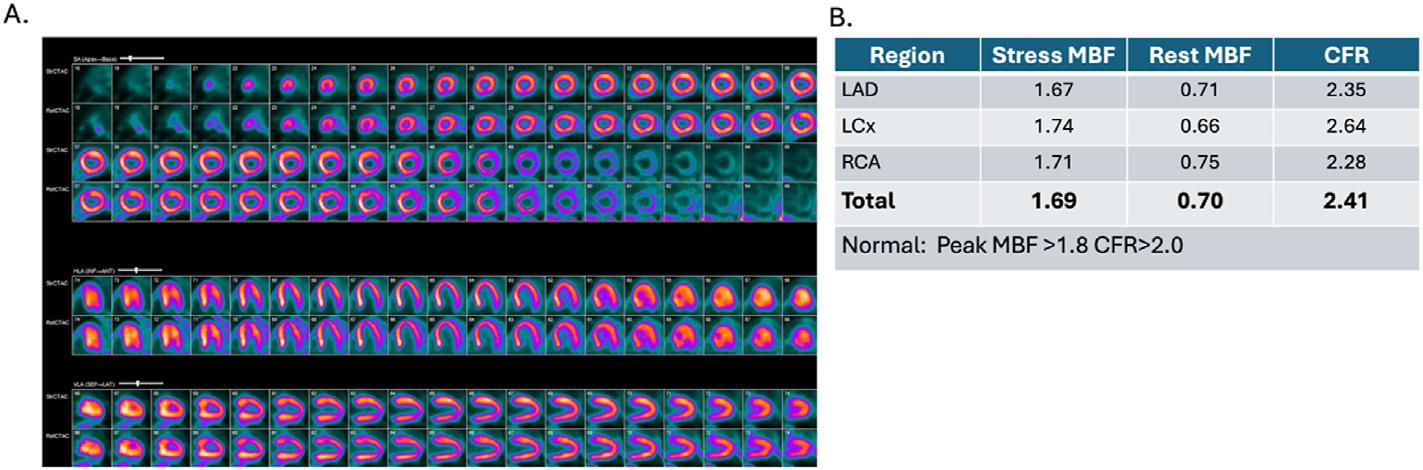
A 62-year old female with GCA in remission on immunosuppressive therapy with chest discomfort. Coronary microvascular dysfunction in a patient with GCA vasculitis in remission. A. ^13^N-ammonia perfusion PET. Perfusion images demonstrate the absence of stress-induced ischemia with vasodilator administration. Transmission CT scan revealed the absence of any coronary artery calcifications (not shown). B. Myocardial flow reserve demonstrates globally reduced peak myocardial blood flow (normal >1.8) with a preserved resultant MFR of 2.41. In the absence of flow limiting ischemia or any coronary artery calcification detected by CT, these results demonstrate diffuse mild coronary vasomotor dysfunction. GCA, giant cell arteritis; MFR, myocardial flow reserve; CT, computed tomography.
